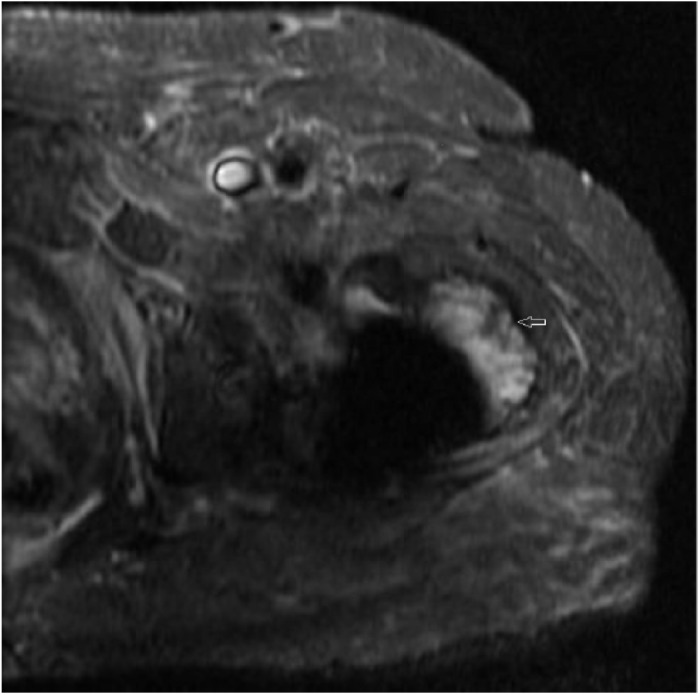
Contrast-enhanced axial T1-weighted MRI image of hip region showing a
